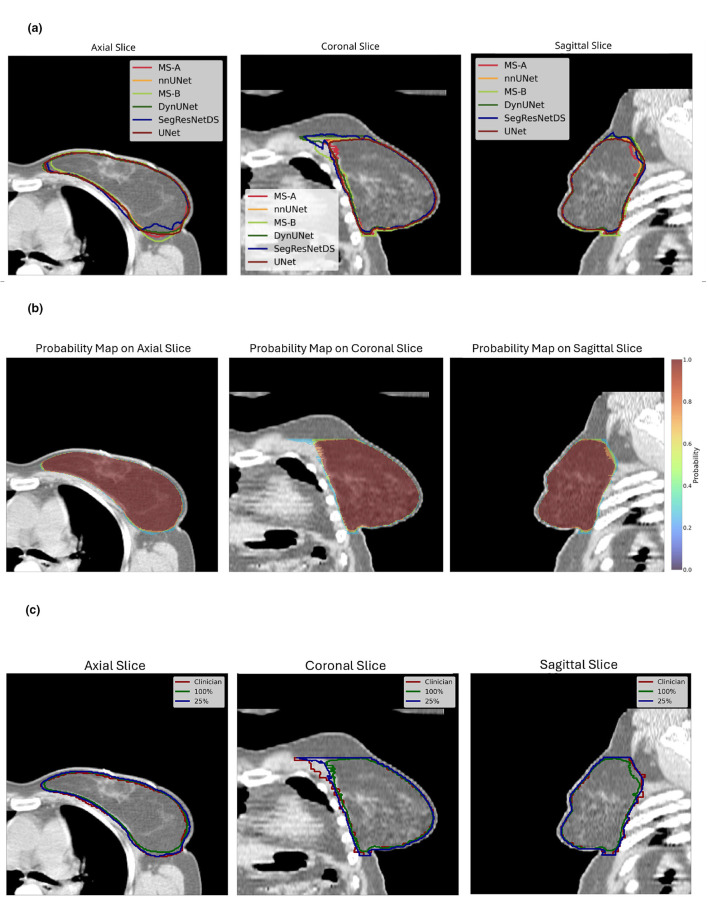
Left breast CTV example across three planes. (a) Six model predictions comparison without cranial caudal cropping. MS-A is MedSAM2-A, MS-B is MedSAM2-B. (b) Probabilistic map (rainbow colormap) derived from cranial caudal cropped predictions of the four best performing models (UNet, DynUNet, nnU-Net, and MedSAM2-A) shown on top of the corresponding CT. (c) Isoprobability contours derived from the probabilistic map with cranial caudal cropping applied. Clinical CTV (red) compared with model-derived isoprobability contours at 25% (blue) and 100% (green).
